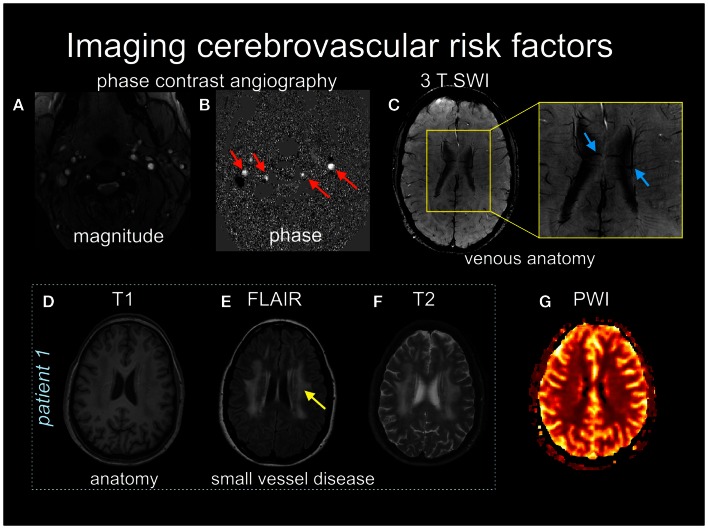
Multiple MRI pulse sequences can be used to assess cerebrovascular risks. The magnitude (A) and phase (B) images in phase contrast angiography can be used to calculate cerebral blood flow velocity in arteries (red arrows) and veins. (C) Susceptibility-weighted images (SWI) provide high spatial resolution (<1 mm in plane resolution) of venous anatomy. The inset shows medullary draining veins (blue arrows). (D–F) Patient 1 provides structural images, T1 (D), FLAIR (E), and T2-weighted (F), with the latter two images showing subcortical white matter disease (yellow arrow). (G) a normal perfusion-weighted image obtained by contrast-enhanced dynamic susceptibility contrast.
